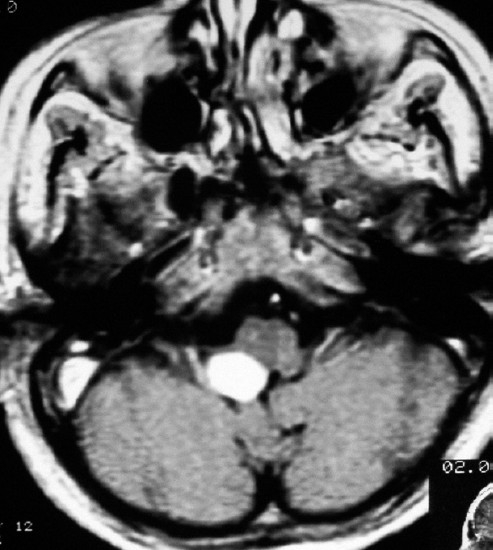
Cranial axial MRI with contrast showing a 1.5 × 2 cm nodular tumor, localized in the right lateral recess of the forth ventricle and showing homogenous enhancement.
